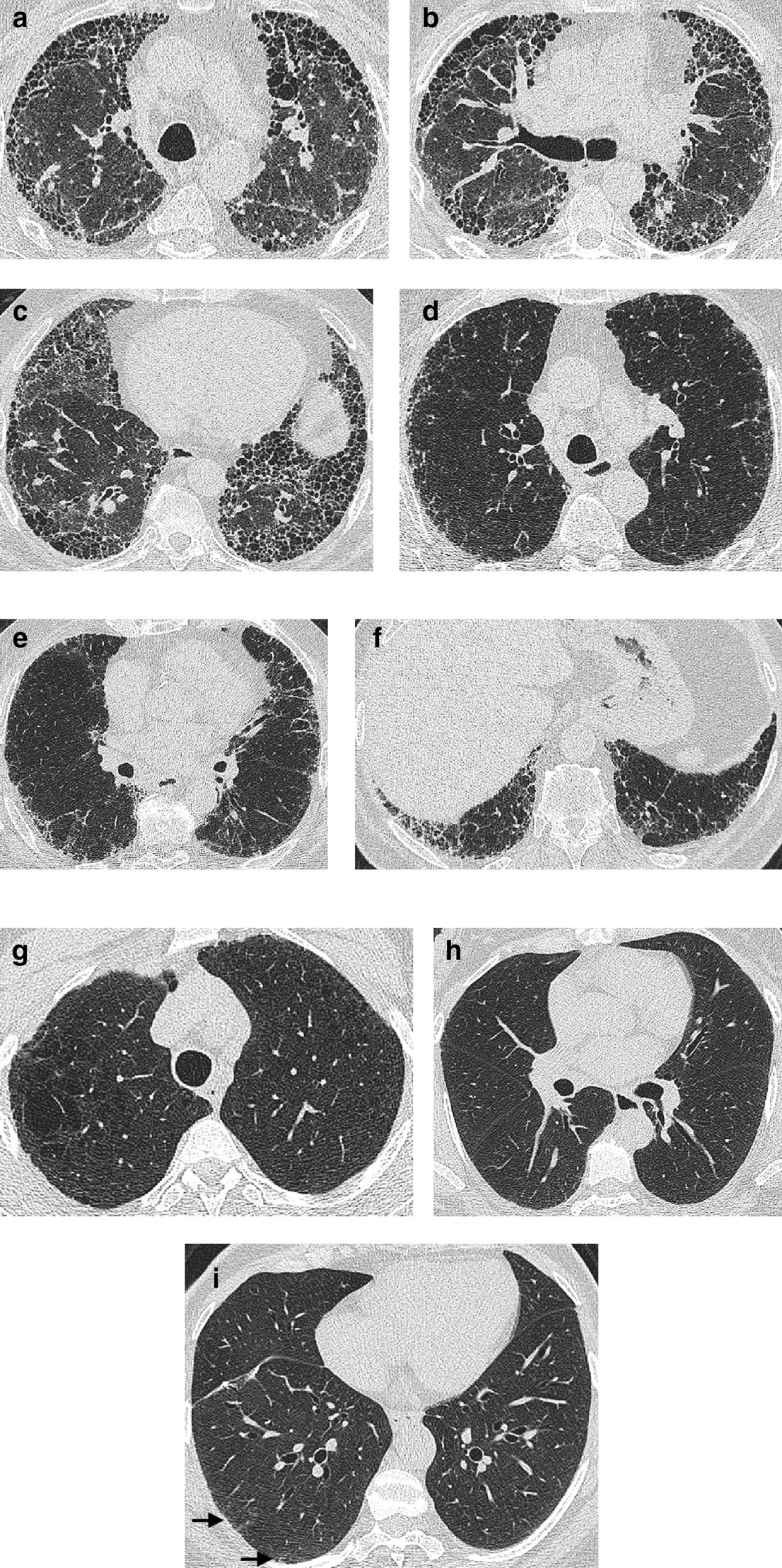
Images of the upper, middle and lower zones of the lung in a patient with a CT pattern of UIP (a–c), probable UIP (d–f) and indeterminate for UIP (g–i). A UIP pattern is characterized by a peripheral basal predominant distribution of disease with honeycombing, with no features suggestive of an alternative diagnosis. A probable UIP pattern is characterized by a peripheral basal predominant distribution of disease with traction bronchiectasis and no honeycombing, and no features suggestive of an alternative diagnosis. Subtle reticulation (arrows) in the right lower lobe and no associated traction bronchiectasis suggests a CT pattern indeterminate for UIP (i). UIP, usual interstitial pneumonia.
